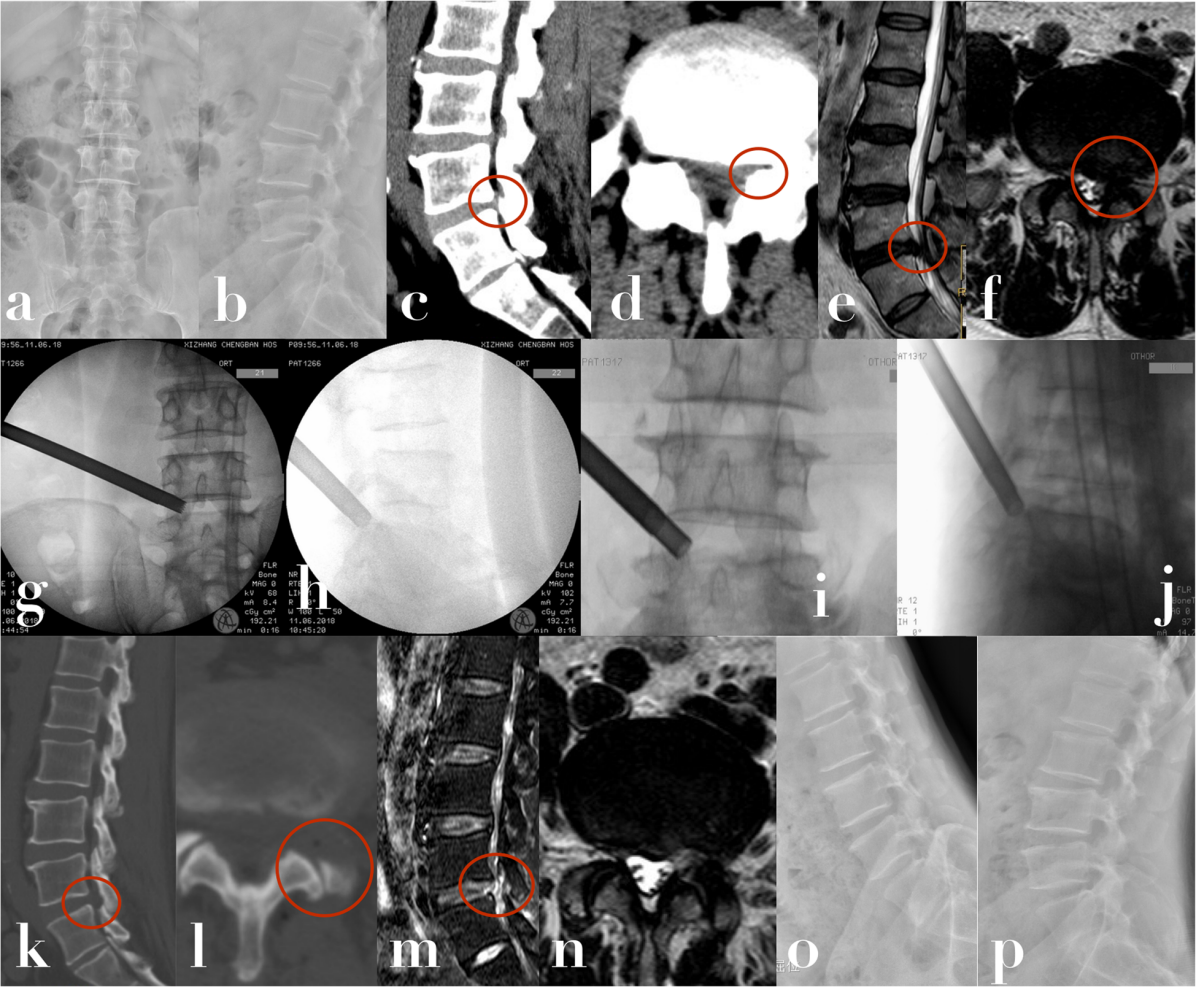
The patient complained of severe left radicular pain for 12 months. He could not walk for 3 months due to severe left buttock and leg pain. Left L4/5 LLSC stenosis in both zones 1 and 2 was confirmed. We confirmed the totally decompression by postoperative CT and MRI. The leg pain was relieved immediately after the operation. No lumbar instability was indicated in the final follow-up. a, b Preoperative X-ray in AP and lateral view. c Preoperative sagittal CT scans indicated stenosis of the retrodiscal space (zone 1) on the left at L4/5 (red circle). d Preoperative axial CT scans indicated upper bony lateral recess (zone 2) stenosis on the left at L4/5 (red circle). e, f Preoperative sagittal and axial T2-weighted MRI scans showing the L4/5 left zone 1 stenosis caused by a lumbar disk bulge anteriorly, and hypertrophied, curled ligamentum flavum posteriorly (red circle). g, h Fluoroscopy during surgery demonstrates the trajectory of the trephine in the first foraminoplasty in the AP and lateral views. i, j Fluoroscopy during the operation shows the trajectory of the trephine in the second foraminoplasty in the AP and lateral views. k, l Three months postoperative sagittal and axial CT bony-window scans clearly demonstrate complete decompression of zones 1 and 2. m, n Three months postoperative sagittal and axial T2-weighted MRI scans indicate that the nerve root was decompressed without recurrence. o, p Postoperative flexion-extension x-rays at the final follow-up confirm that no lumbar instability occurred
